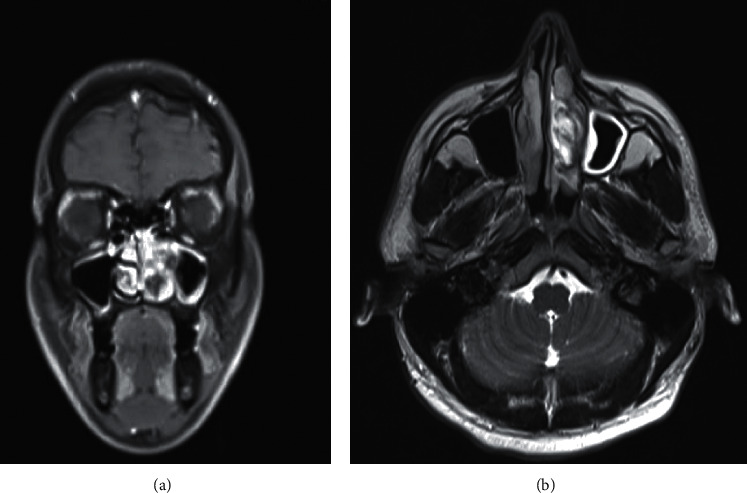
MRI scan of the paranasal sinuses with contrast. The coronal and axial section shows a left anterior heterogeneous hypointense nasal mass.
